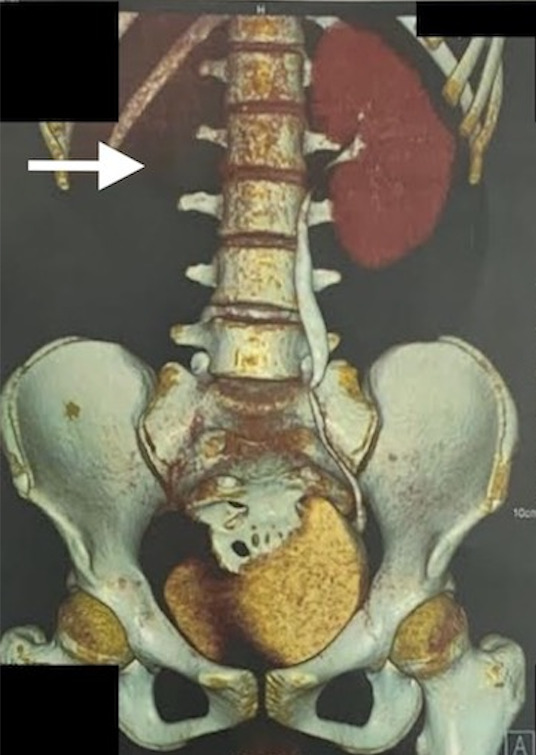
CT urogram with 3D reconstruction showing absent right kidney and ureter.
